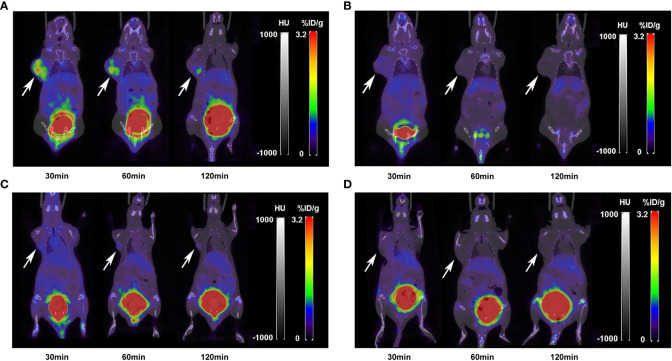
Micro-PET/CT imaging of (A) RT-112, (B) A549, (C) SNU-16 and (D) Calu-3 xenografts injected with 11.1 MBq [^18^F]F-FGFR1 at different time points. [^18^F]F-FGFR1 revealed tracer uptake in the kidney, bladder and RT-112 (FGFR1-high expression) cell-derived tumors. Arrows indicate tumors.
